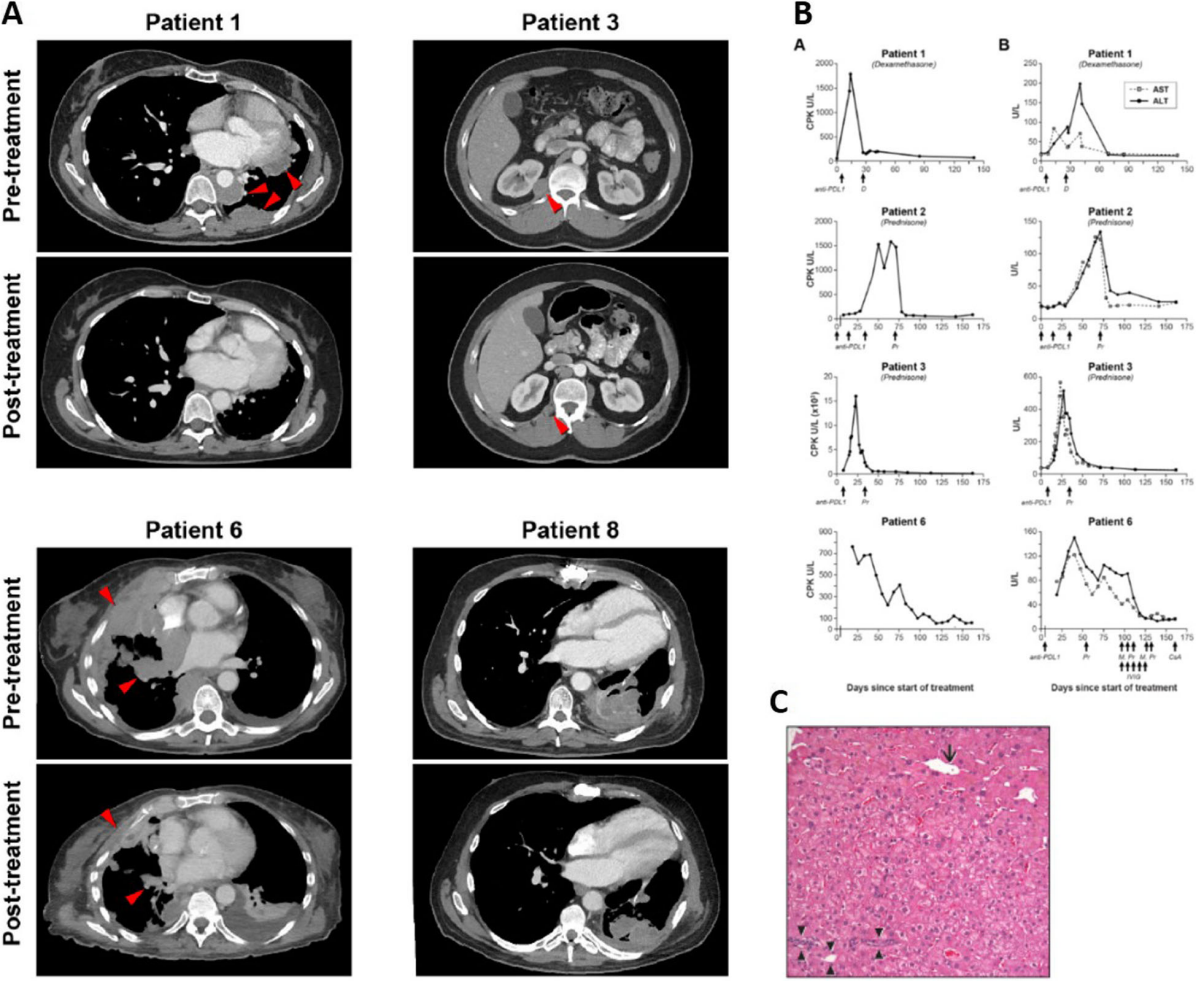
Changes in radiographic appearance of tumor and laboratory parameters after treatment. a Changes in selected target lesions in patients responding to treatment. Representative axial CT images of patients achieving a partial response to treatment showing the maximum change in size of selected tumor lesions. b Biochemical changes in response to treatment with avelumab. Column A, on the left, shows changes in CPK. Column B, on the right, shows changes in AST and ALT. Three out of four patients (1, 3, and 6) also developed a radiological response to treatment. In these three cases, only one dose of avelumab could be administered due to the development of autoimmunity. Days of administration of avelumab and other medications are indicated by arrows. c Post-treatment liver core biopsy from patient 1 with portal space (arrowheads), centrilobular vein (arrow) and no evidence of inflammation. CPK: creatinine phosphokinase, AST: aspartate transaminase, ALT: alanine transaminase, D: dexamethasone, Pr: prednisone, M.Pr: methylprednisolone, IVIG: intravenous immunoglobulin, CsA: cyclosporine A
